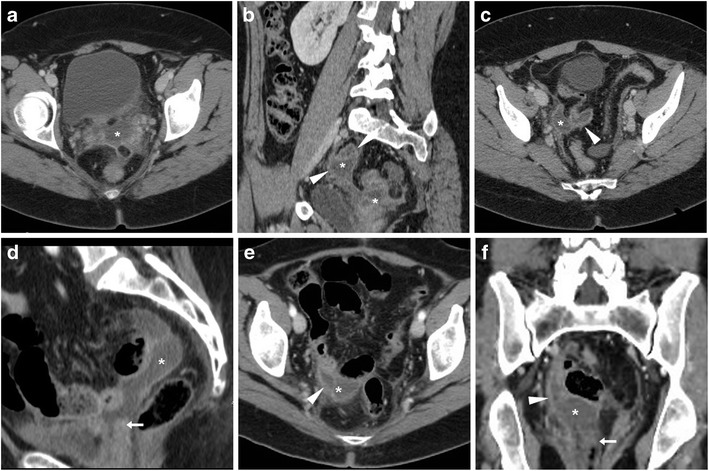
Multidetector CT appearances in two cases of surgical site infections. A–C) after recent laparoscopic hysterectomy (LH), clinical diagnosis of pelvic cellulitis corresponded to a bilobated fluid-like collection (*) with thin peripheral enhancement. D–F) after radical AH, a larger abscess (*) with peripheral enhancement (arrowheads) developed upwards from the normal-appearing vaginal dome (arrows) (Partially reproduced from open access ref. no [58])
